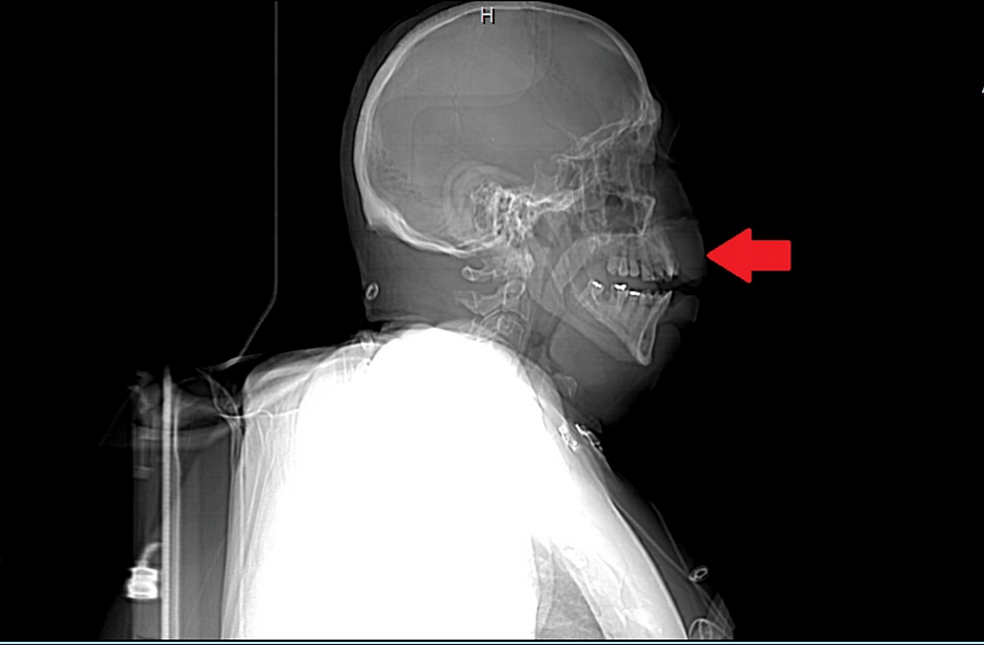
CT neck sagittal view showing angioedema of upper lip. The red arrow highlights the edema present in the upper lip.
